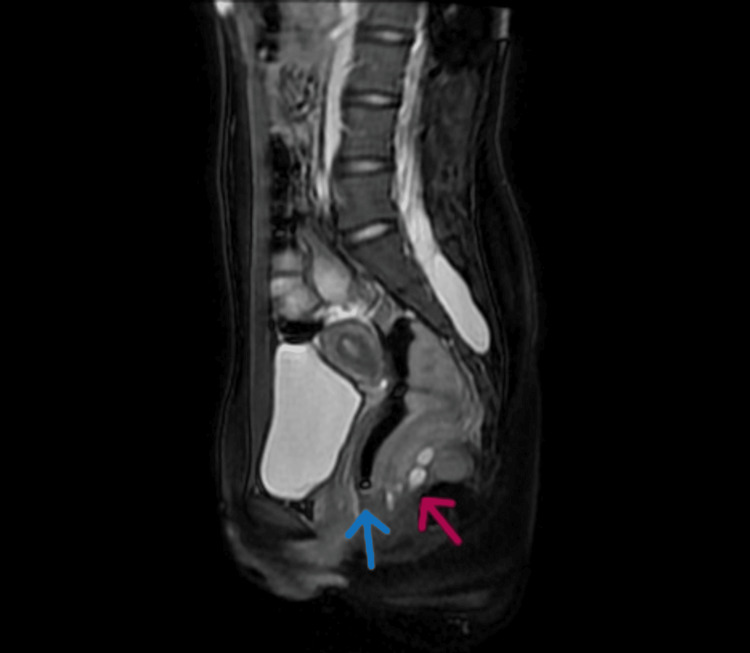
Sagittal 2D FIESTA MRI view demonstrating a well-defined perirectal collection with central necrosis, suggestive of perirectal abscess formation localized within an anal accessory canal (red arrow), without communication with the principal canal (blue arrow) 2D FIESTA MRI: two-dimensional fast imaging employing steady-state acquisition magnetic resonance imaging
